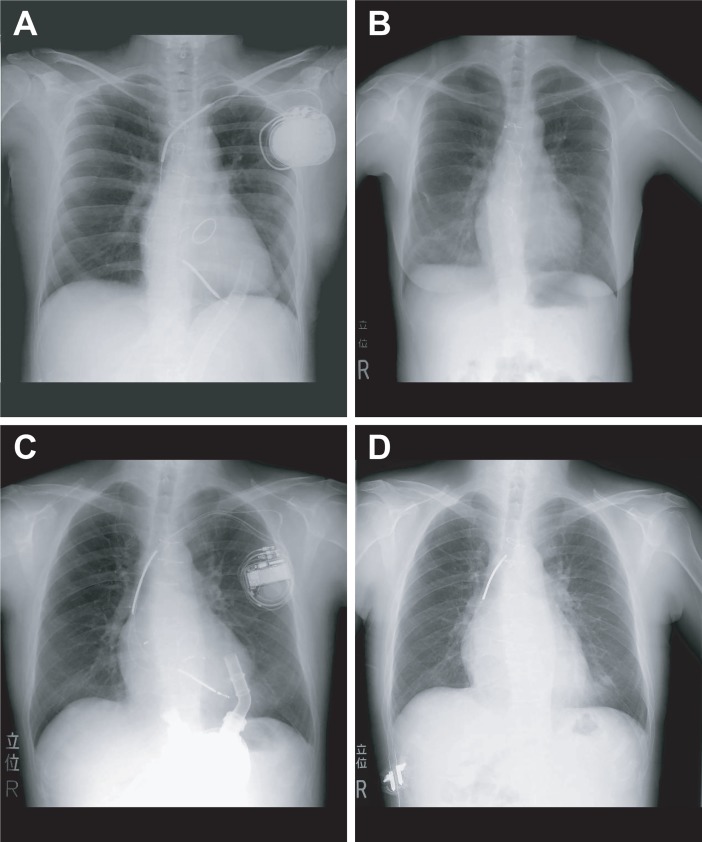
The patients with the retained fragment in superior vena cava after OHT. (A) Chest X-ray of a patient who had an ICD with dual coil shock lead before OHT, (B) Lead fragment from a dual coil defibrillator in the patient shown in (A), as observed in the subclavian vein after OHT. (C) Implantation of a CRTD with dual coil shock lead. (D) Retained distal shock coil in superior vena cava after OHT.
